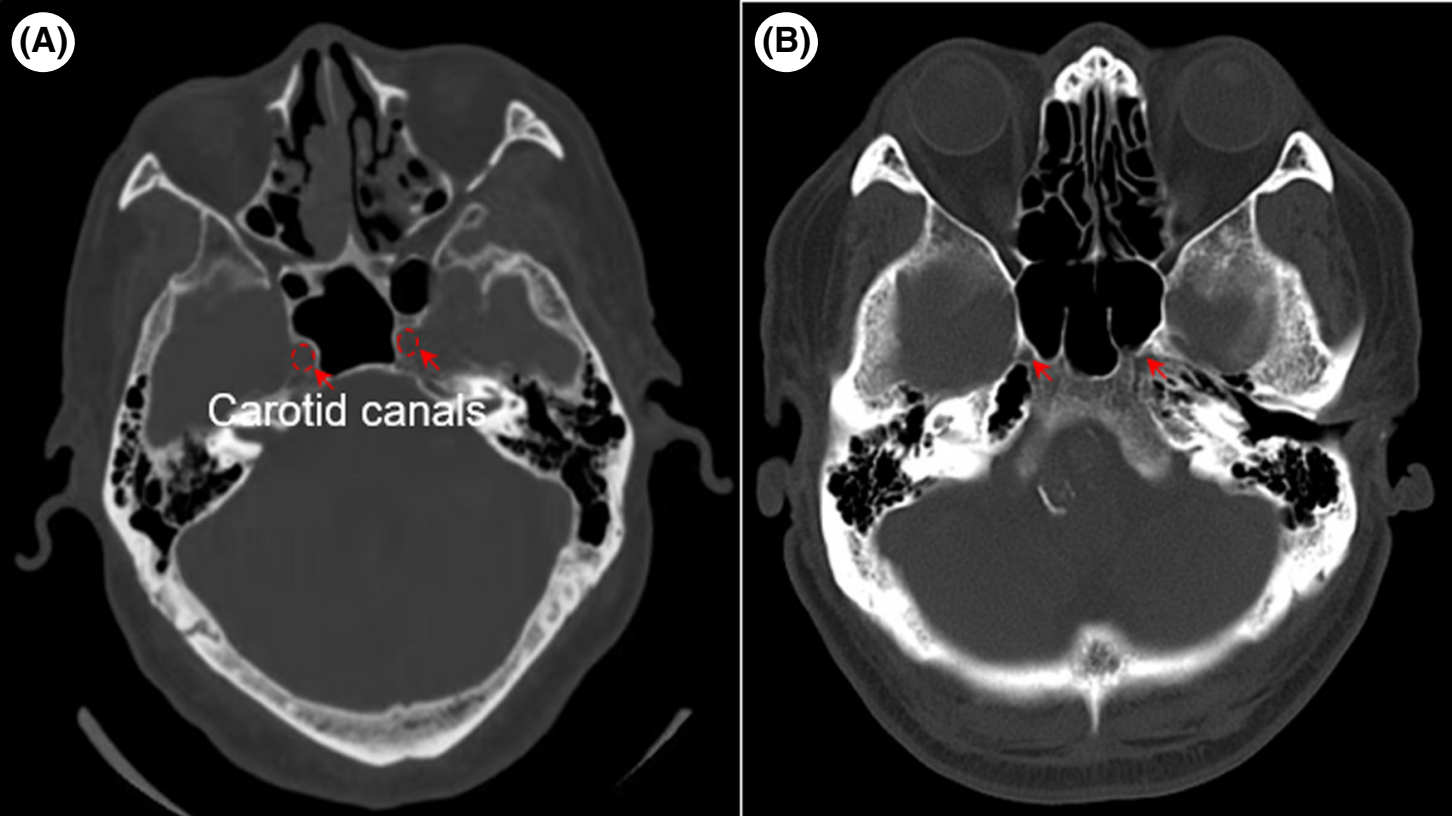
CT scan reveals hypoplastic carotid canals at both sides in the patient. (A) The ICA enters the skull through the carotid canals (red circle) in healthy control, (B) while the patient had none.
